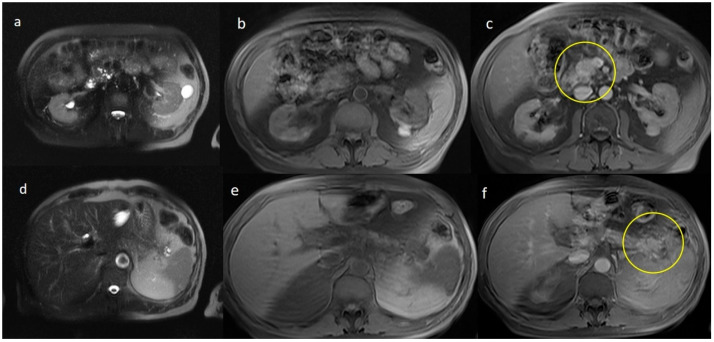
Imaging findings in the same patient with synchronous pancreatic ductal adenocarcinoma as depicted in Figure 1. (a,d) MRI T2WI with fat saturation: lobulated masses displaying intermediate signal intensity and ill-defined borders. (b,e) T1WI with fat saturation and (c,f) T1 with gadolinium and contrast enhancement (venous phase) showing inhomogenous enhancement of the masses and fat stranding (Yellow circles).
